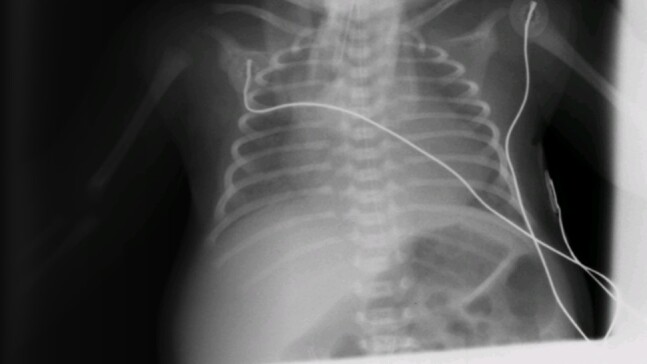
Chest x-ray of the patient. General thinning of the bones are presented on the chest x-ray done shortly after his birth.
